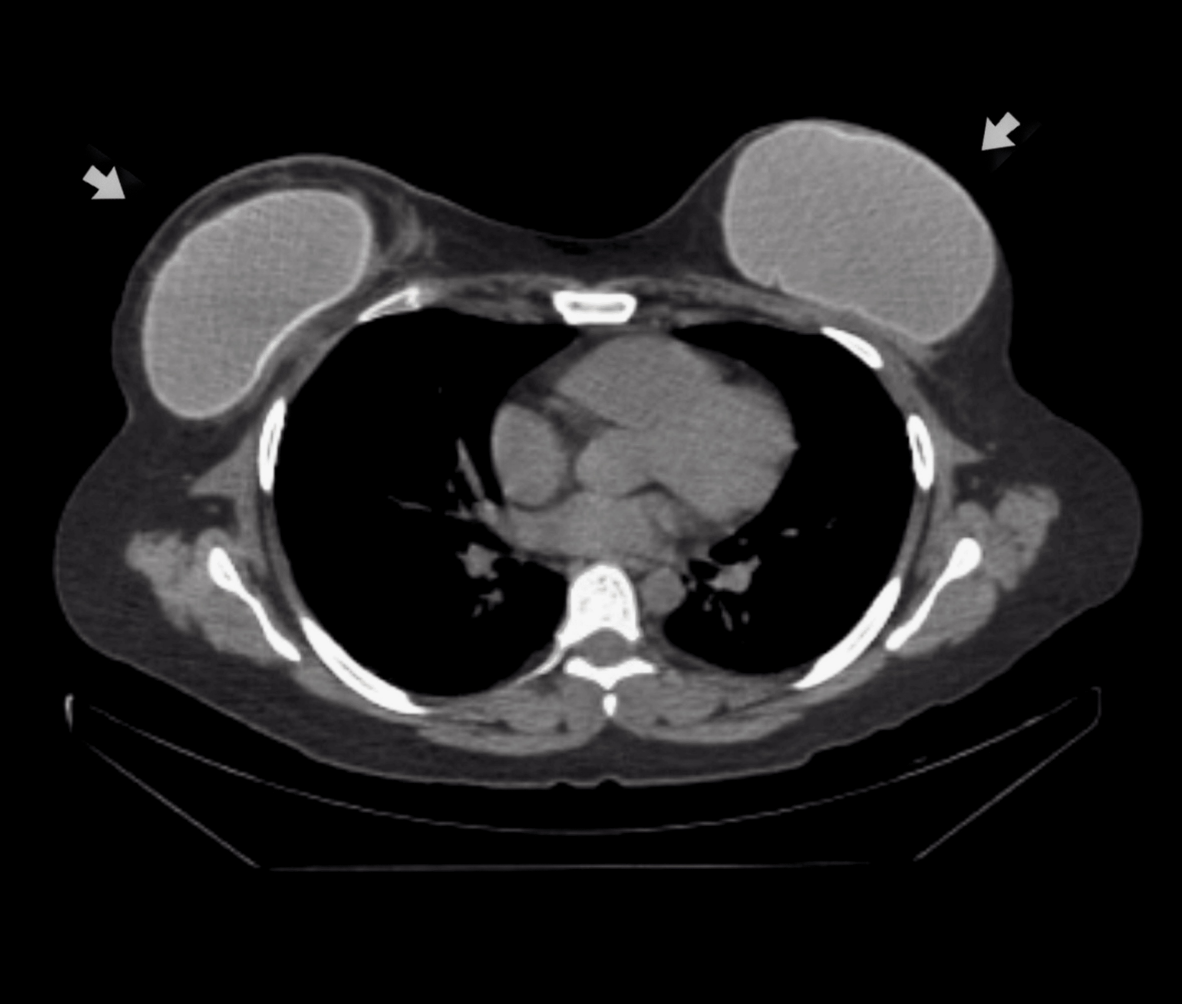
Implants that are intact in a CT: The implants are indicated by both arrows with their capsule preserved, well positioned and without signs of rupture or migration CT: computed tomography
